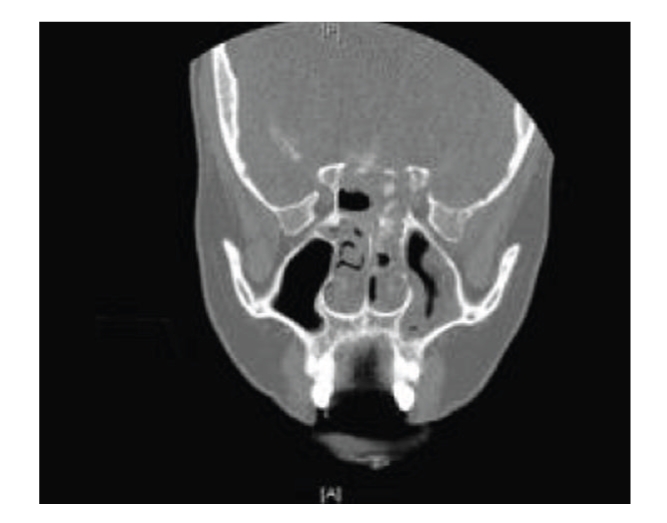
CT cisternogram showing a right-sided CSF leak through the roof of the sphenoid sinus.
